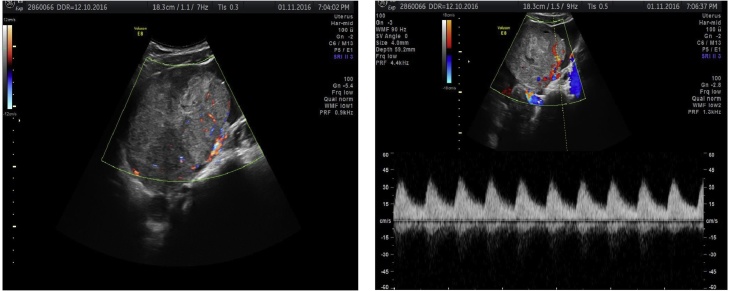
The abdominal ultrasound showes a left ovarian heterogeneous and high vascularized mass of 13 cm long axis and a moderate amount of ascites.
